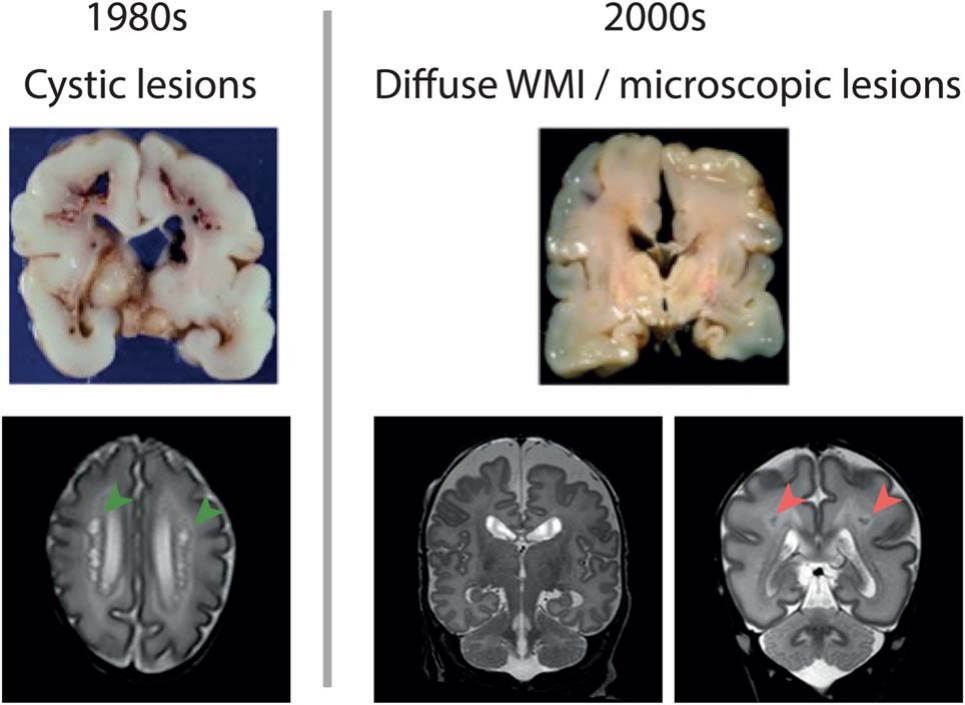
The clinical problem of perinatal white matter injury (WMI) has evolved over time. Upper panels: photographs of postmortem brain slices of preterm infants with WMI (published with permission from http://neuropathology-web.org/). Lower panels: T2‐weighted MR images of preterm infants with WMI. Left part of the figure: in the 1980s, cystic periventricular leukomalacia (cPVL) was often observed in preterm infants. cPVL is associated with large cystic lesions in the white matter clearly present at macroscopic postmortem tissue and at (transverse) MRI scan (T2 sequence) as indicated by the green arrowheads. cPVL leads to severe disabilities such as cerebral palsy. Right part of the figure: at present, diffuse WMI is most often associated with atrophy of white matter causing loss of brain volume (middle (coronal) MRI scan) and punctate white matter lesions (PWML) (right (coronal) MRI scan: red arrowheads). Diffuse types of WMI are mainly associated with impaired cognitive functioning later in life
